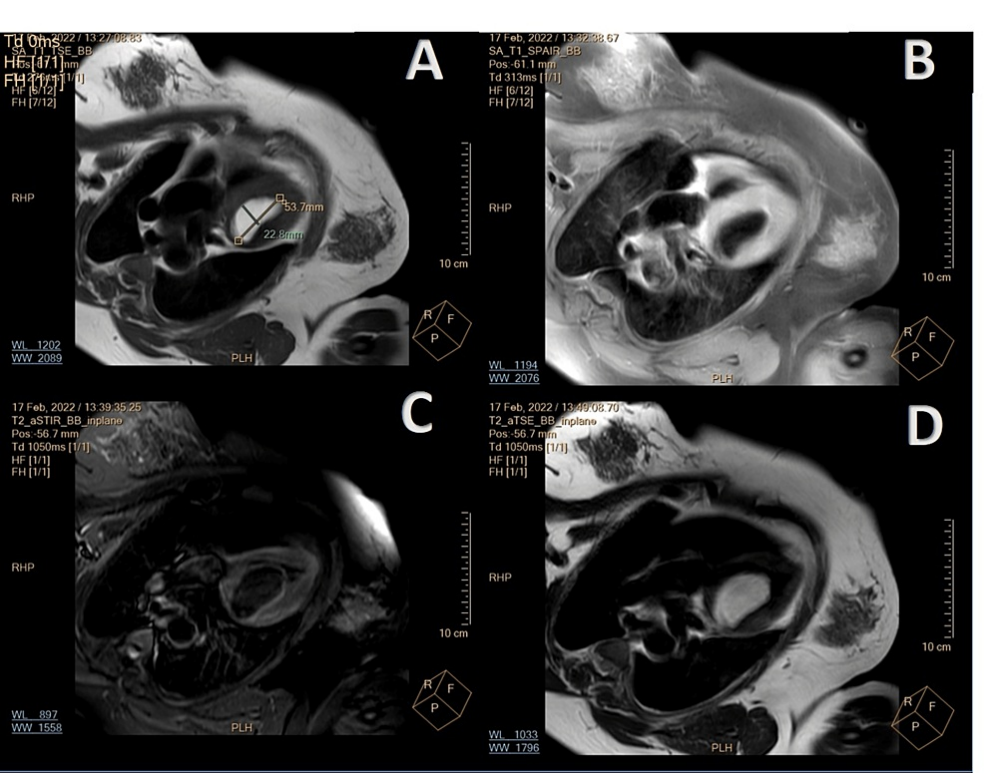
MRI of the mass (A) Panel T1 turbo spin echo (TSE) and (B) TSE with fat saturation. Notice the lack of signal on the fat saturation images. (C)  Panel demonstrates SSFP image of the mass and (D) hyperintense signal of the tumor on T2-weighted images. SSFP, steady-state free precession
